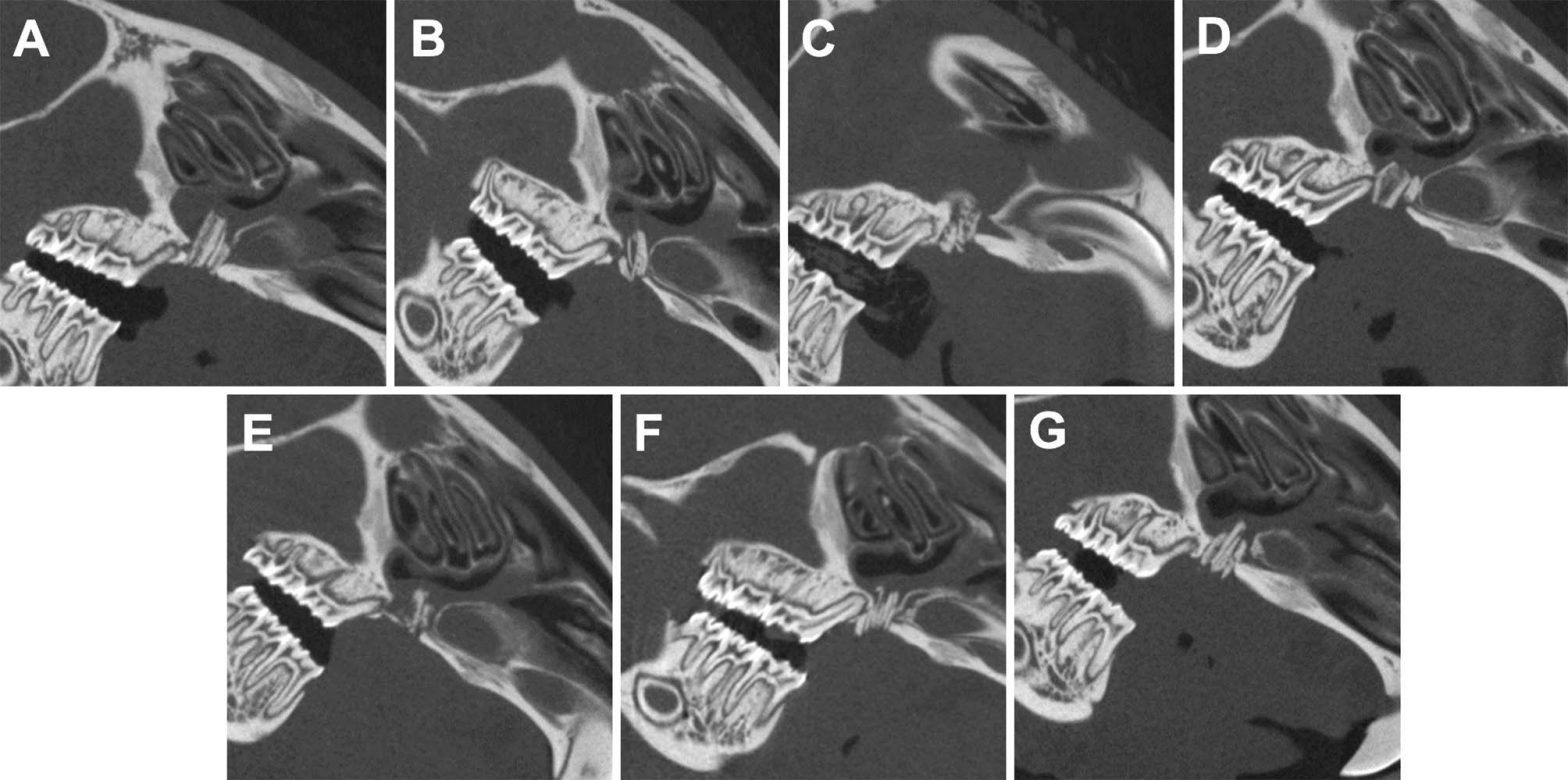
Sagittal view of all CT scans two days after cleft repair demonstrating an over contouring into the nasal cavity of varying degrees. Figures were generated with Imalytics Preclinical software 2.1 (Gremse-IT, Aache, Germany) ^25^.
